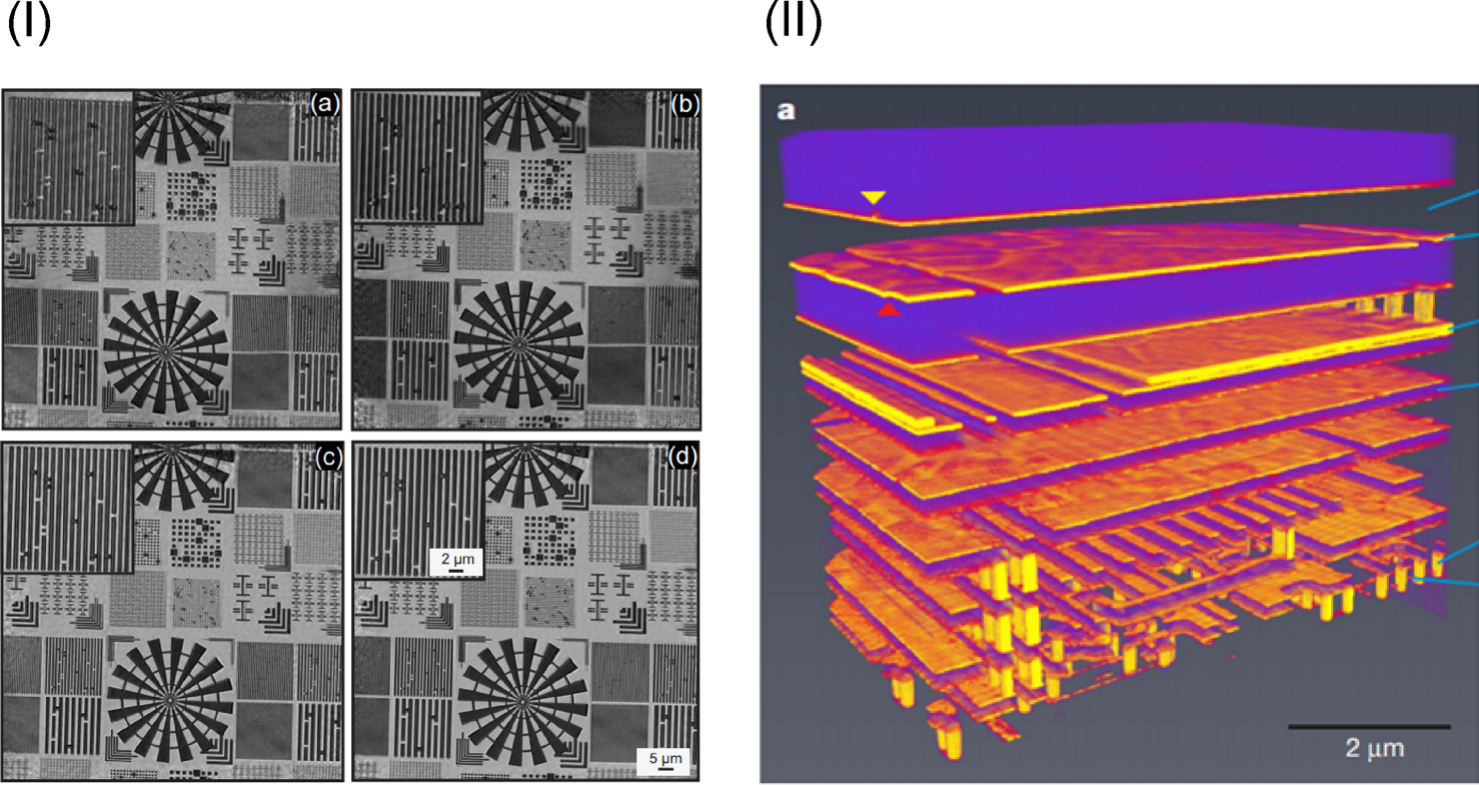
Example applications for the use of lensless imaging methods in the XUV/SXR-regime. (I) Reflection ptychography at an energy of 92 eV from a synchrotron with a different number of probes and modes of the beam. (a) 1 mode, 1 probe (b) 1 probe, 3 modes (c) 40 probes, 1 mode, and (d) 40 probes, 3 modes. Reprinted with permission from Helfenstein *et al.*, Opt. Express 26, 12242 (2018). Copyright 2018 OSA. (II) Ptychographic 3D-X-ray-tomography of the application of a specific integrated circuit of a hybrid silicon pixel detector at an energy of 6.2 keV at a synchrotron. Reprinted with permission from Holler *et al.*, Nature 543, 402–406 (2017). Copyright 2017 Authors, licensed under a Creative Commons Attribution 4.0 License.
